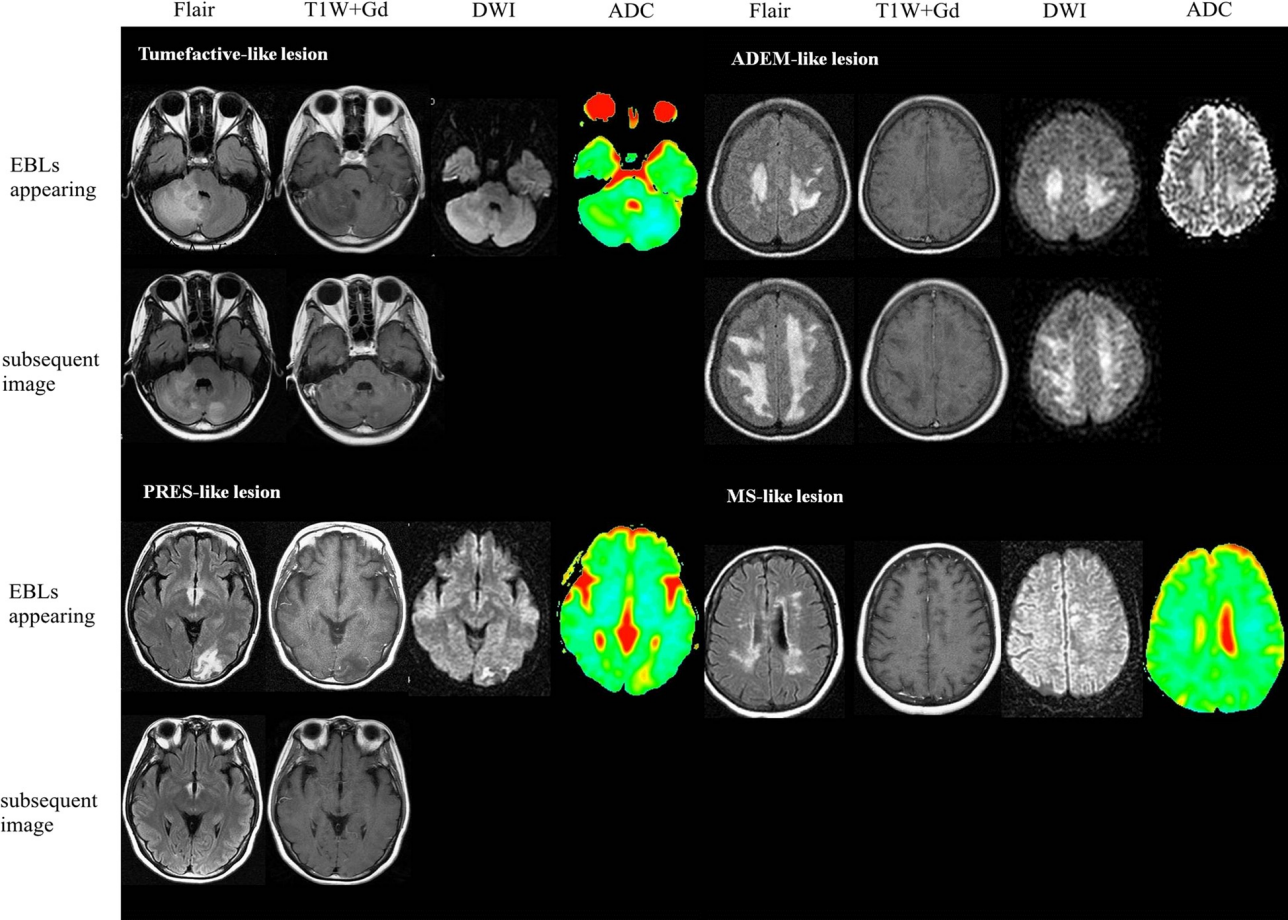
Typical four typies images of EBLs are displayed according to the following MRI sequences, as available: fluid-attenuated inversion recovery (FLAIR), T1W+Gd, diffusion-weighted imaging (DWI), apparent diffusion coefficient. The top rows represent neuroimaging at time of EBLs appeared, and the bottom rows represent post-EBLs repeated neuroimaging. Tumefactive-like lesion: No enhanced tumefactive-like lesions in cerebellar hemisphere shrink in repeating imagings of two months later. ADEM-like lesion: No enhanced extensive bilateral white matter brain lesions are markedly expanded in the subsequent MRI of three months later. PRES-like lesion: No enhanced lesions in left occipital lobe completely resolve in less than four weeks. MS-like lesion: No enhanced multifocal lesions locate in the periventricular and subcortical white matter. The MR images of follow-up are not supplied. The DWI, ADC images and MR images of follow-up are not supplied. All the DWI and ADC images reveal changes consistent with vasogenic edema.
